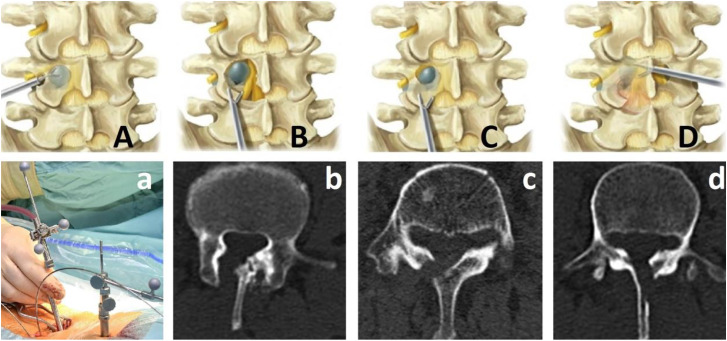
Possible approaches for endoscopy and spondyloscopy (for implant-supported segmental restoration) in the lumbar spine for reduced surgical access modalities: (a) navigation guided endoscopy sleeve for transforaminal approach (A). (b) CT scan after endoscopic interlaminar approach (B). (c) CT scan after spondyloscopic translaminar approach (C). (d) CT scan after spondyloscopic crossover translaminar approach (D).
